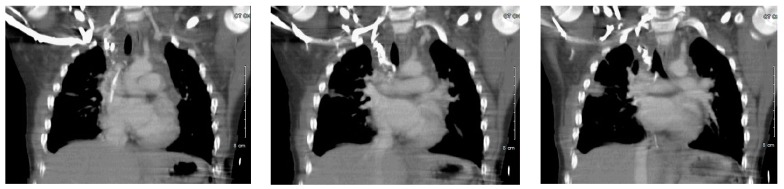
Chest computed tomography (CT) (intravenous (IV) contrast) scan demonstrating inability to fully visualize the superior vena cava (SVC) in total, and no opacification of the right internal jugular (IJ). Images from left to right demonstrate CT slices in an anterior to posterior fashion, respectively.
